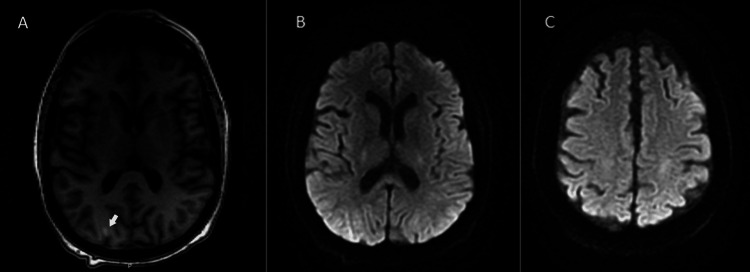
Brain MRI with and without contrast DWI axial images one week after prior imaging (A) New T1 gyriform cortical enhancement within the right occipital lobe corresponding to the area of DWI diffusion restriction found in Figure 1B; (B, C) interval resolution of hyperintensities previously seen in Figures 1B, 1C.
